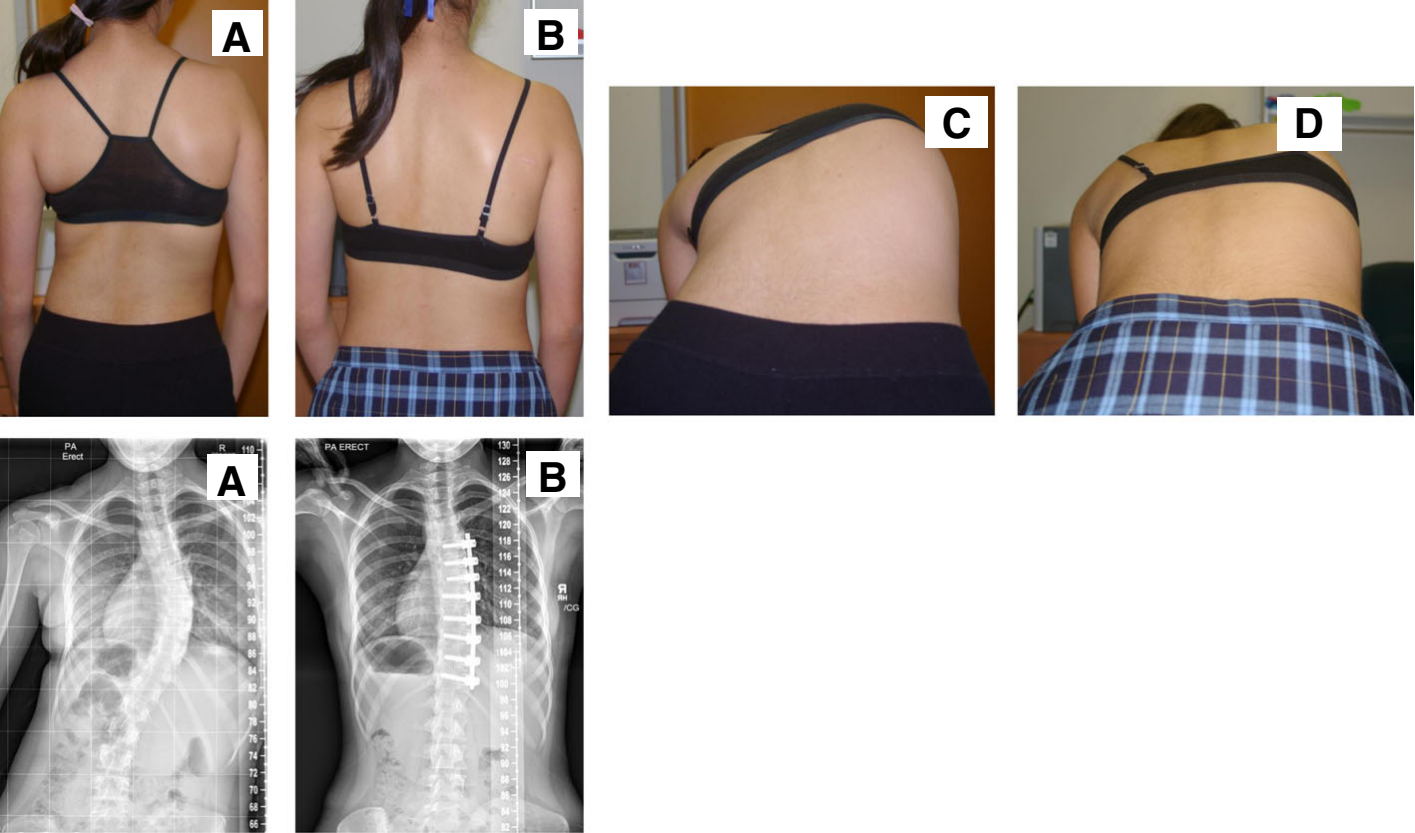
Photographs and radiographs of a typical TASF case (a) standing before and (b) standing after surgery, (c) forward bending before and (d) forward bending after surgery
